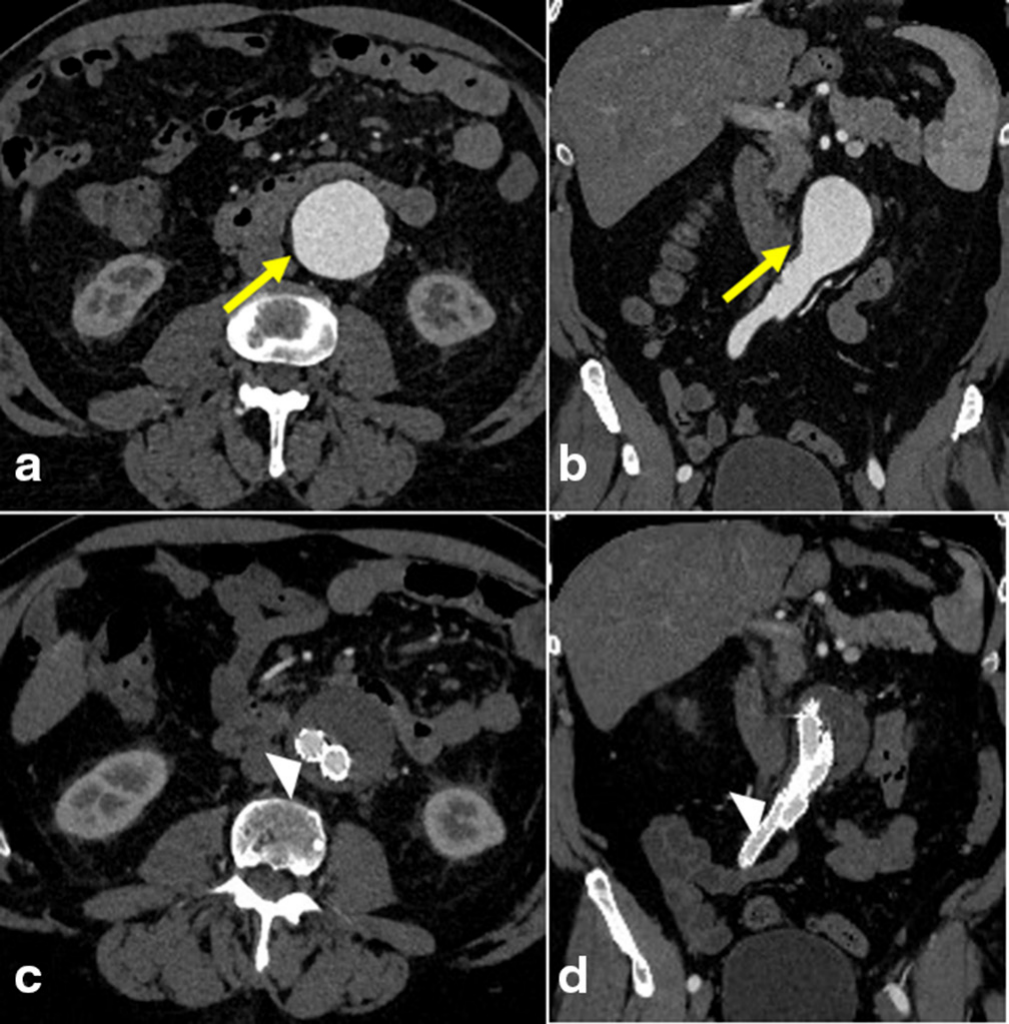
CT angiogram at the time of presentation. Axial (a) and coronal (b) images show a 4.7- × 4.5 cm infrarenal abdominal aortic aneurysm (arrow). Axial (c) and coronal (d) images show an aorto-bi-iliac endovascular stent graft (arrowhead). The aneurysm sac is stable in size. There is no contrast opacification of the excluded aneurysm sac on arterial or delayed venous phase images (latter not shown).
